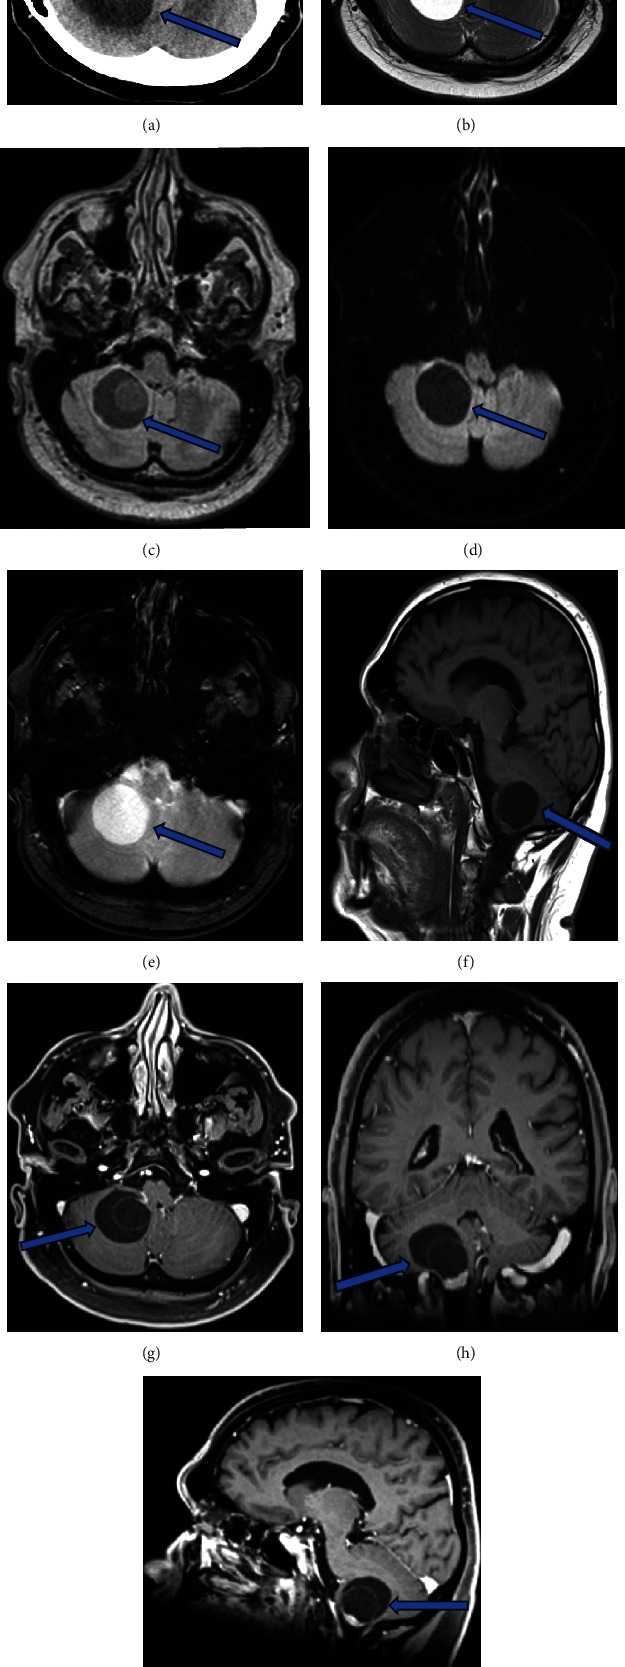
Right intracerebellar cystic lesion. (a) Axial noncontrast head CT shows a simple intra-axial cystic mass within the right cerebellum. The content is mildly heterogeneous. There is mild local mass effect effacing the fourth ventricle and causing slight tonsillar herniation with no vasogenic edema or obstructive hydrocephalus. (b) Axial T2-weighted image confirms the thin-walled cystic nature of the mass but also reveals a cyst-within-a-cyst element. (c) FLAIR demonstrates a hyperintense signal of the cyst-within-a-cyst which indicates slight complexity of the fluid content. (d) ADC MRI reveals no diffusion restriction of the fluid content which excludes epidermoid cyst. (e, f) T1 images lack susceptibility and hyperintense signal which rules out hemorrhage. (g, h, i) 3D T1-post gadolinium images reveal a small nodular component at the inferior aspect of the mass, associated with the cyst-within-a-cyst.
